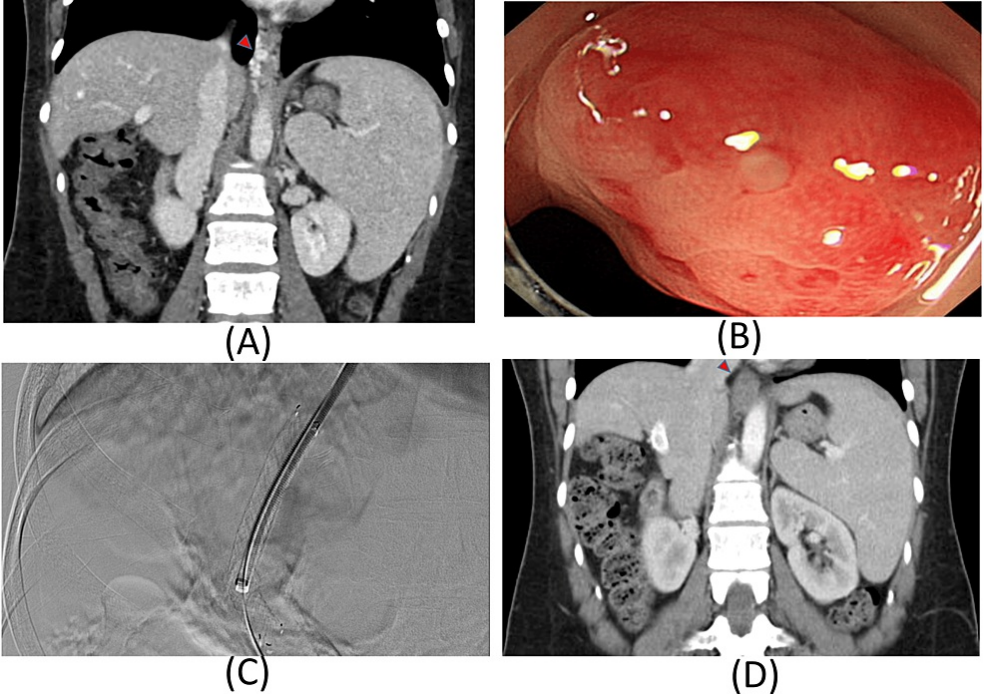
CT scans and intraoperative view of the TIPS procedure A: Coronal view of the CT scan showing splenomegaly and varices but no sign of cirrhosis; B: Esophagogastroduodenoscopy showing esophageal varix with 'white nipple sign' at the gastroesophageal junction; C: TIPS procedure; D: Coronal view of the CT scan showing resolution of previously noted varices with patent TIPS stent MDS: Myelodysplastic syndrome; TIPS: Transjugular intrahepatic portosystemic shunt
